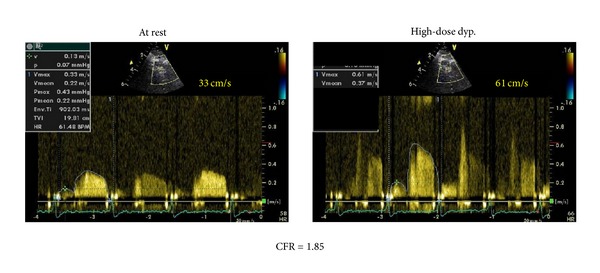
High-dose dipyridamole induced CFR during the same stress echocardiography of Figure 2.
